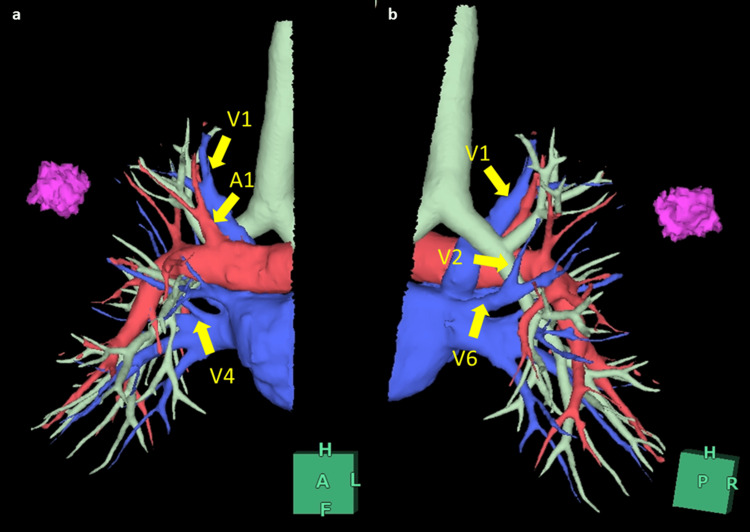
Preoperative estimation of pulmonary vessels and bronchi using three-dimensional computed tomography (a) The anomalous V1 runs behind the superior trunk of the pulmonary artery (A1), and the V4 drains into the inferior pulmonary vein. B3, A3, and V3 are absent, and segment 3 is aplastic. (b) The aberrant V2 drains into the V6 behind the right bronchus intermedius.
